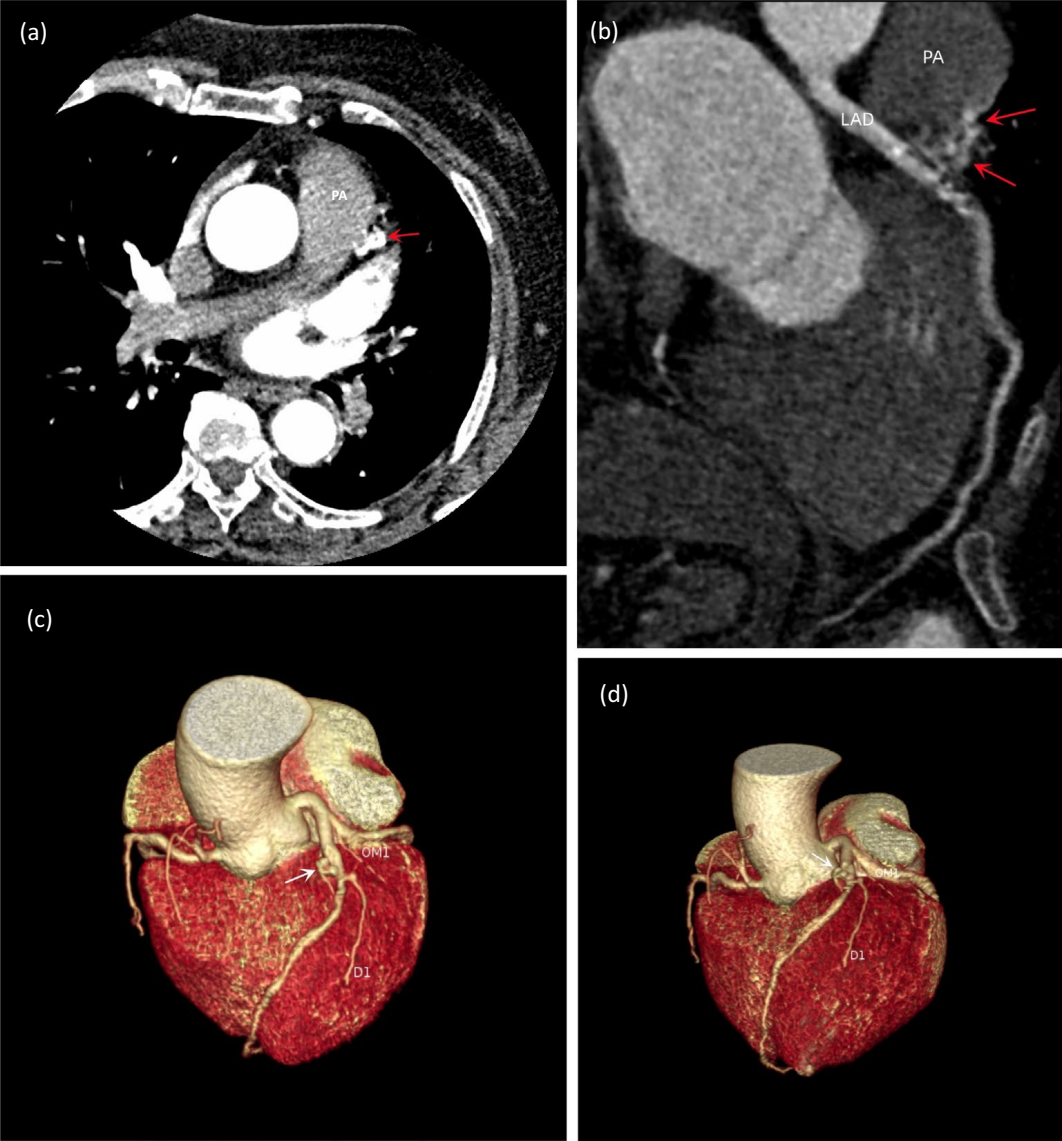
(a) The CT shows an abnormal vascular mass (red arrow) with a hint of high‐density at the PA connection, indicating minor blood flow ejection into PA. (b) Curved reconstruction CT also reveals near the PA, minimal high‐density suggests slight blood flow via the fistula (red arrow). (c, d) The CTA revealed 3D images of the fistula (white arrow), but there was no visualization of the PA. CTA, Computed Tomography Angiography; D1, first diagonal branch; LAD, left anterior descending; OM1, first obtuse marginal branch; PA, pulmonary artery.
